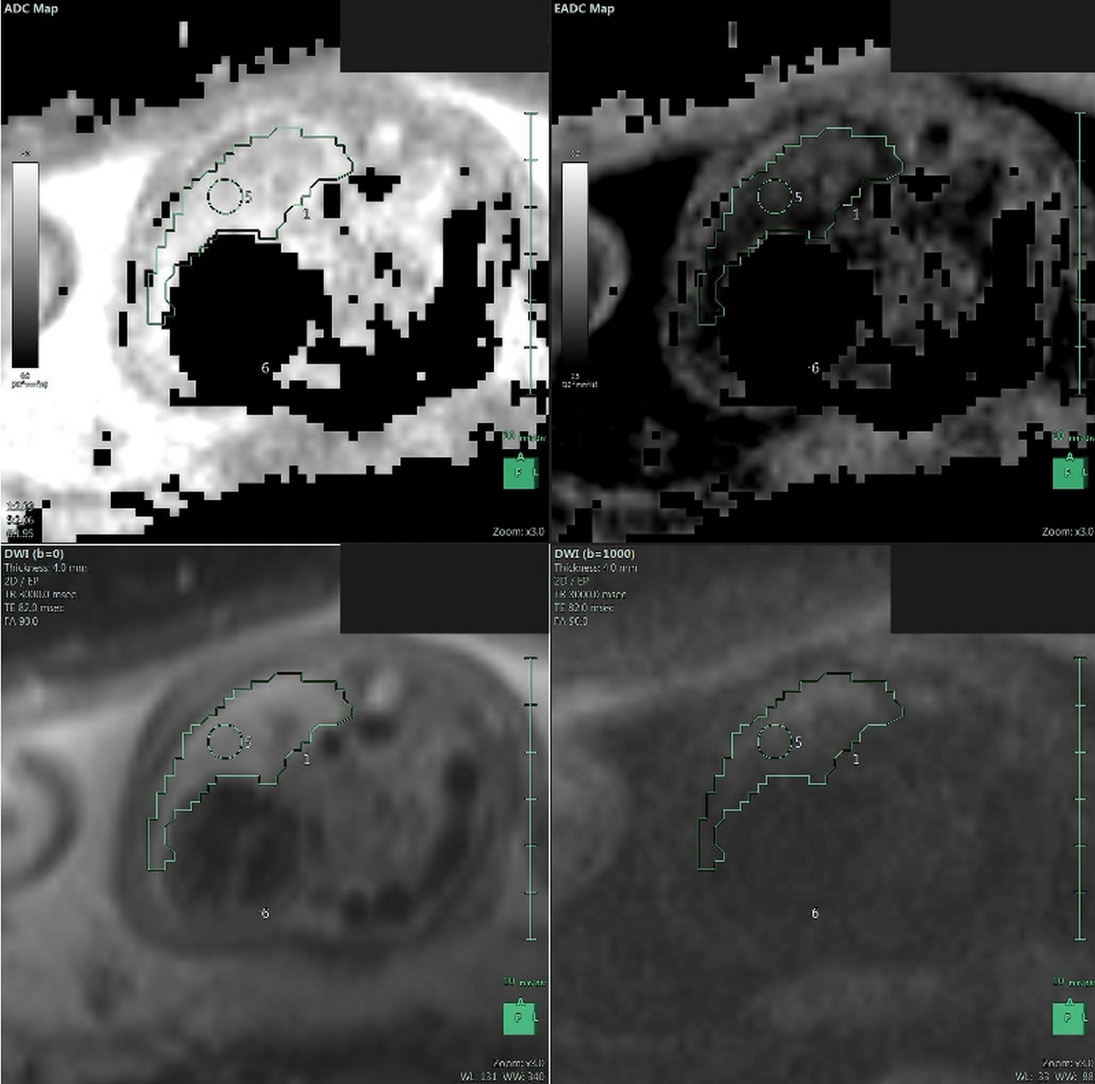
Apparent diffusion coefficient. MRI calculation of the apparent diffusion coefficient (ADC) on diffusion-weighted sequences.
